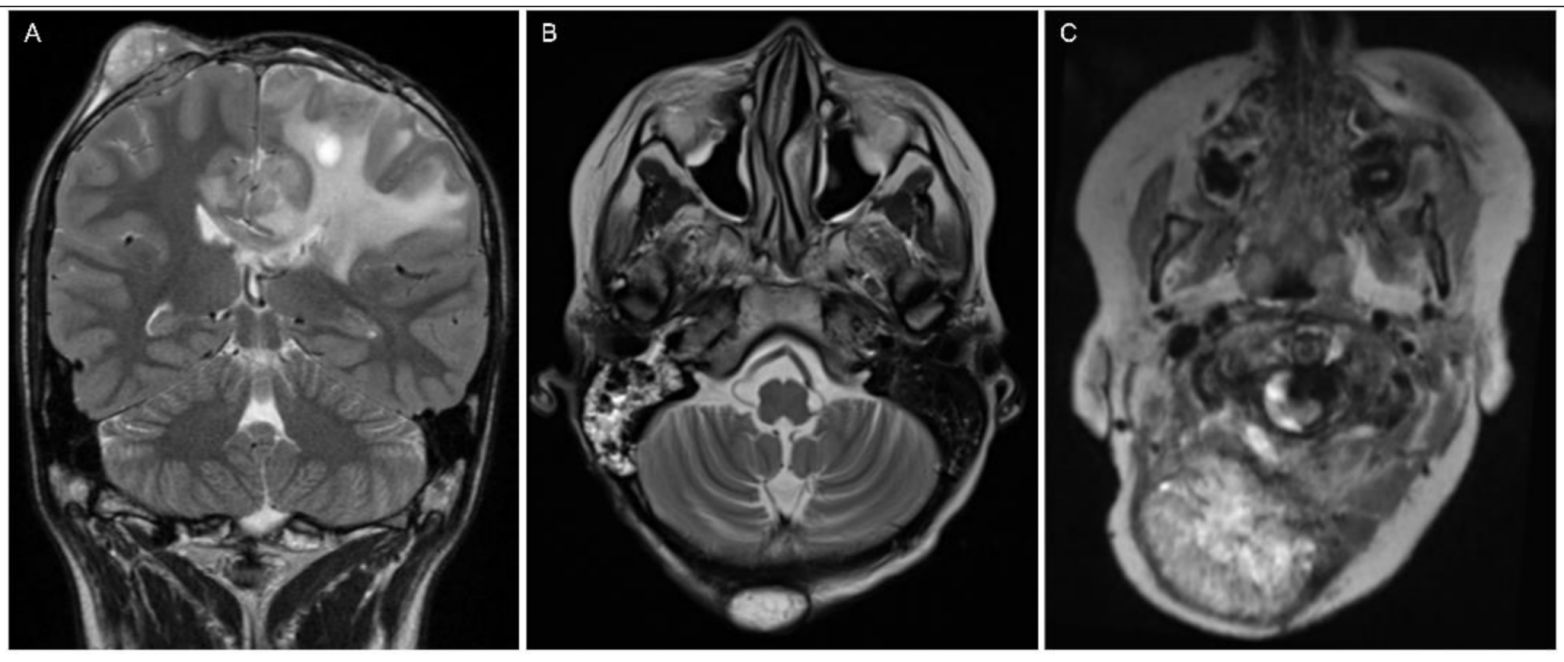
Extraneural metastases on follow-up (T2-weighted images). (A) A male patient diagnosed with a supratentorial ependymoma at the age of 10 developed a new subcutaneous soft tissue lesion near the first surgical access more than four years after the first resection. (B) A female patient diagnosed with a supratentorial ependymoma at the age of 11 developed a new subcutaneous lesion without connection to the surgical access more than four years after diagnosis. Here, no lymph nodes were present on earlier MRI studies. (C) A male patient diagnosed with a posterior fossa ependymoma at the age of 4 developed a large extracranial mass along the way of surgical access six years later
